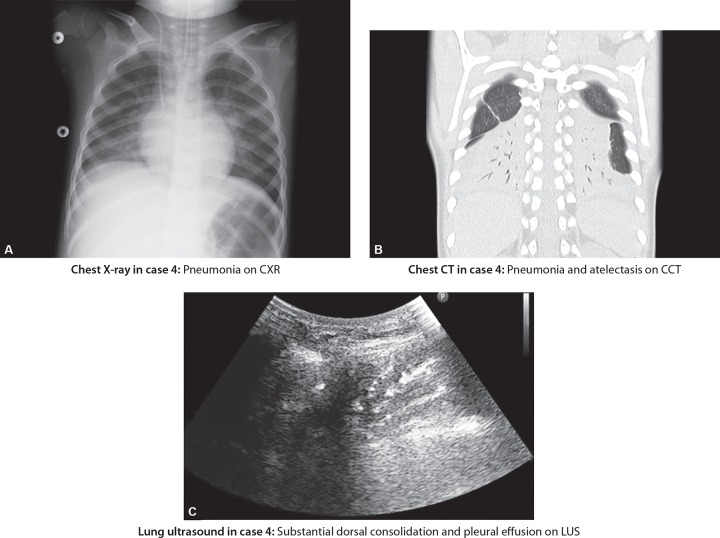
Comparison between chest X-ray, chest CT and lung ultrasound in case 4
